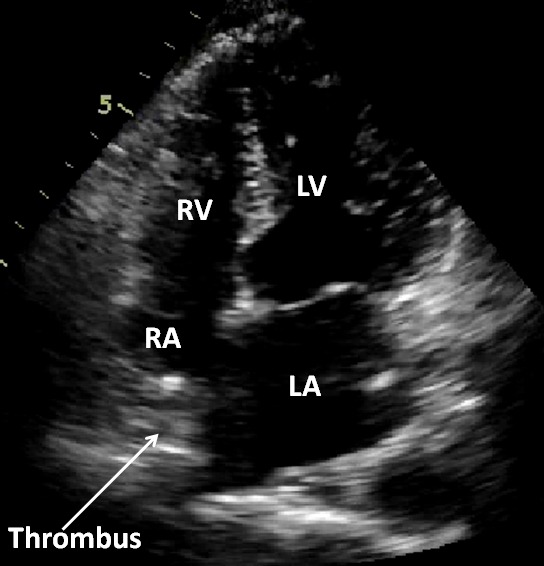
2D Echocardiogram - Apical 4 chamber view showing thrombus in the right atrium
